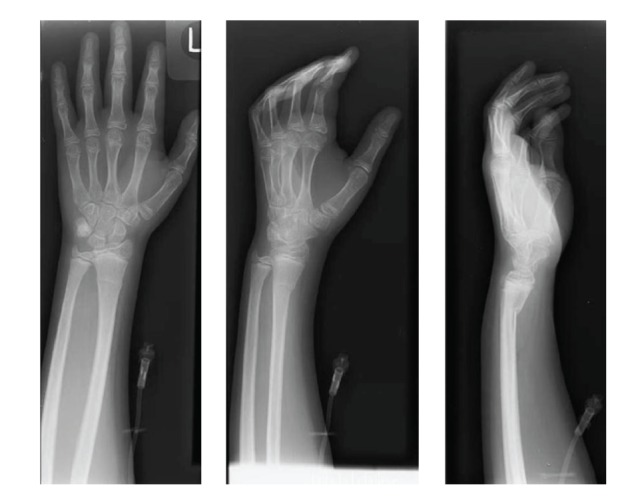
Radiographs of left wrist showing apex-dorsal distal radius buckle fracture.
